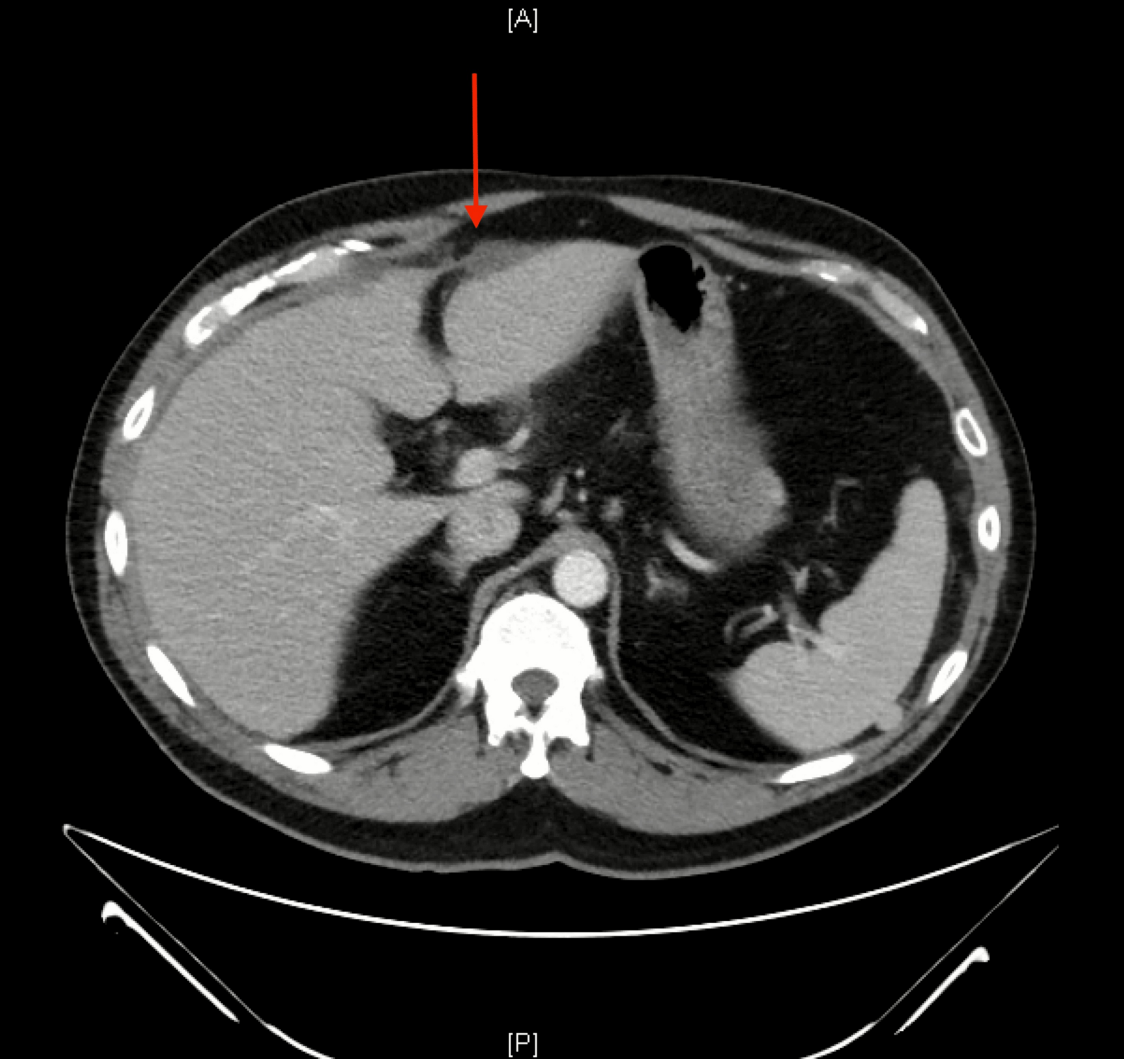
Axial CT scan of the liver The red arrow shows intraperitoneal free fluid anterior to the liver. A: anterior; P: posterior
